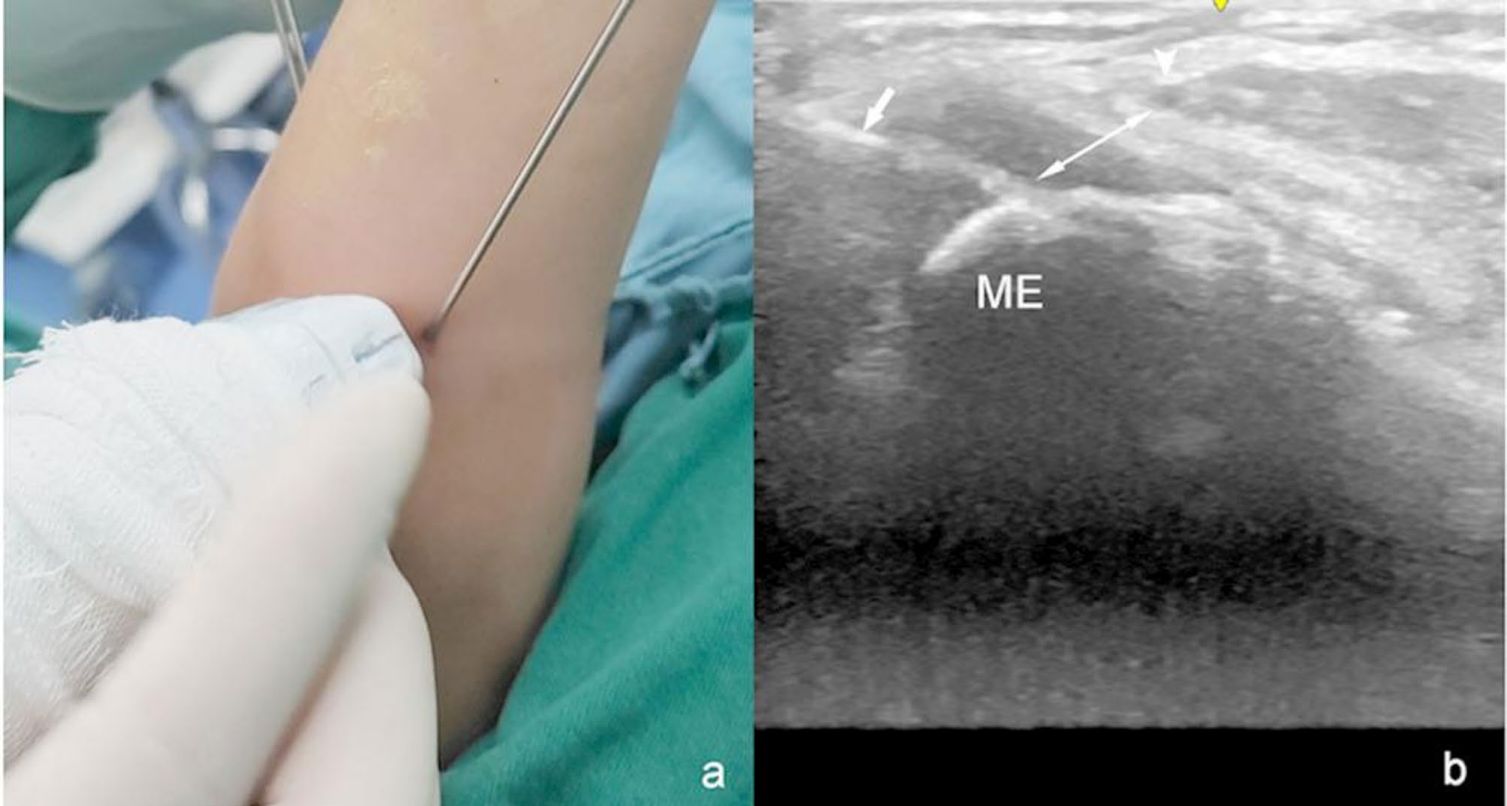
Photographs showing the positions of the oblique transducer (a), and corresponding ultrasound sections (b). Oblique section ultrasound in an elbow extension showing the relationship between the ulnar nerve (arrowhead) and medial pin (arrow) can all be seen. ME: medial epicondyle of humerus; double-ended arrow: the distance between ulnar nerve and medial pin
